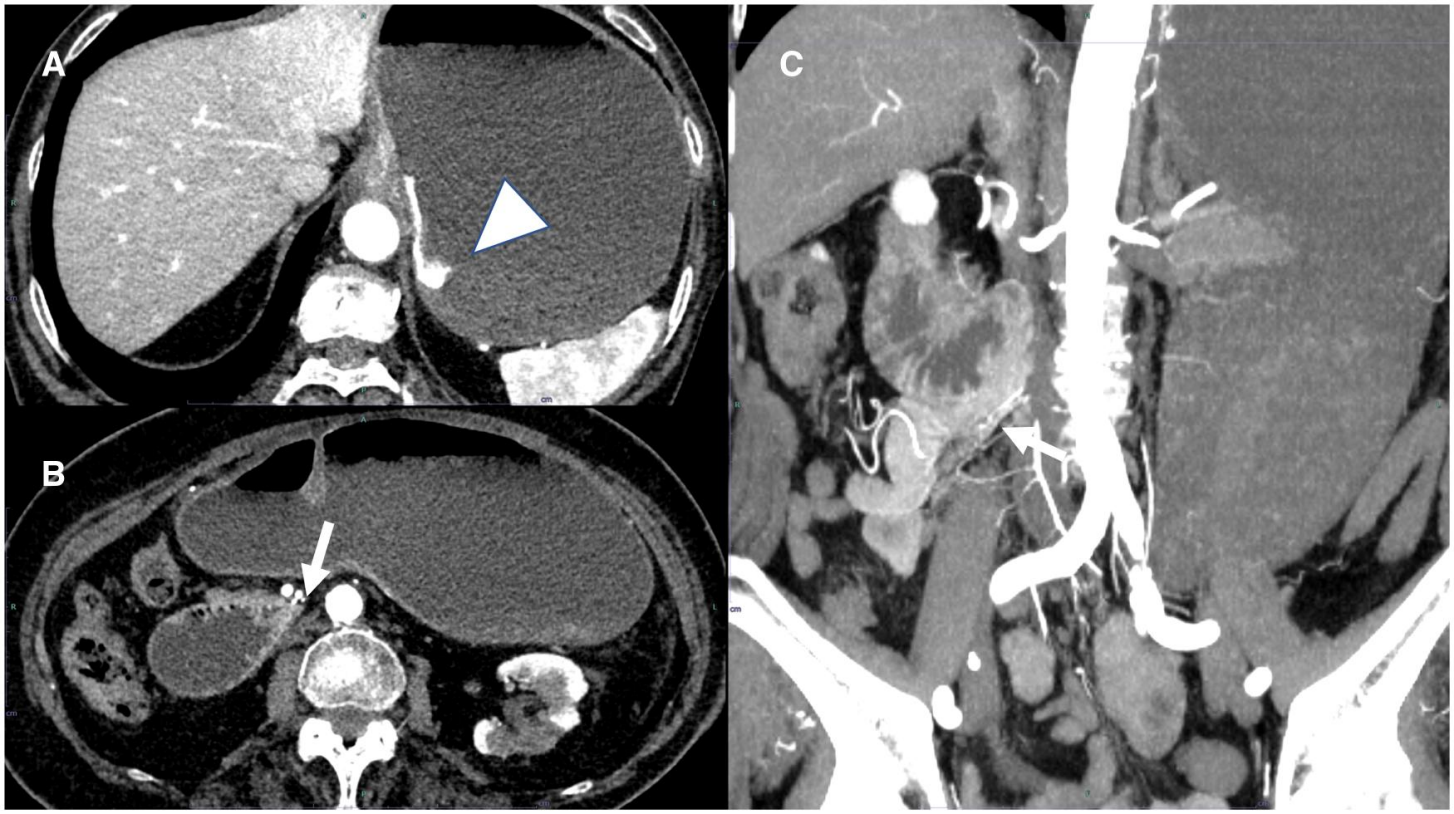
Dynamic CT performed 2 months after the first admission when the patient was rushed to the emergency room for repeat haematemesis. In the arterial phase, the stomach is dilated with fluid, and contrast extravasation from the gastric cardia to the gastric fundus is visible (A, arrowhead). The jejunum is also located on the right side (B, C: arrow).
